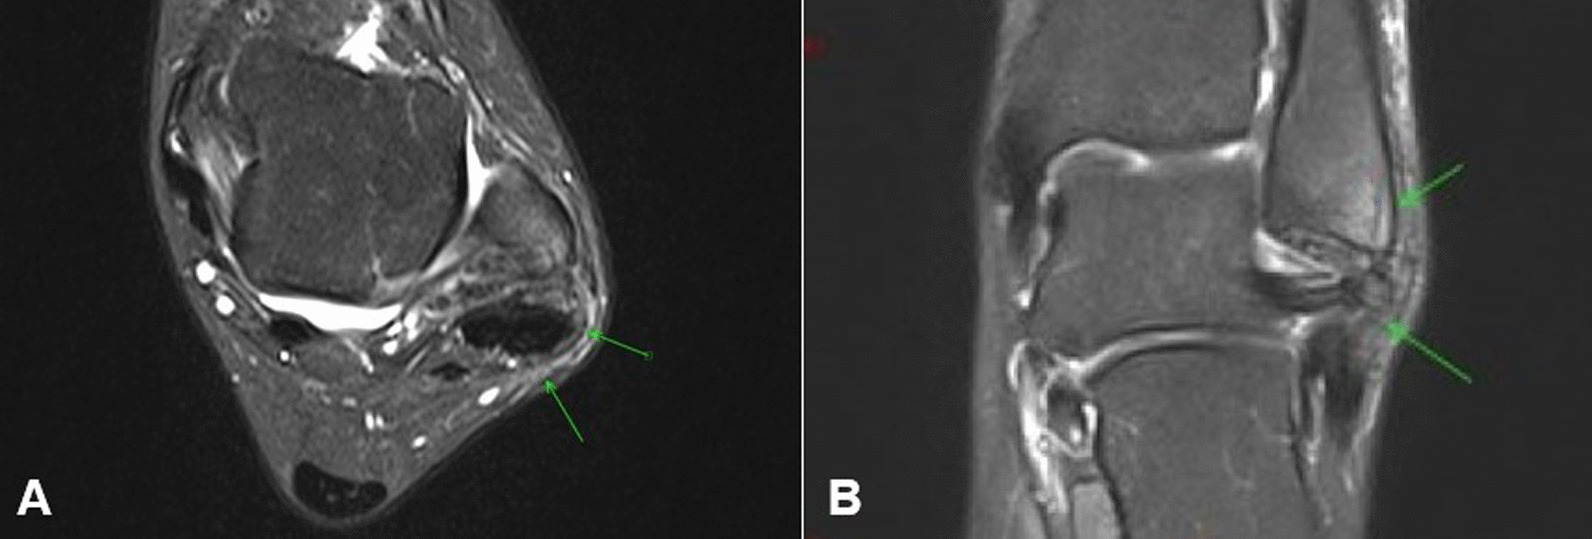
A Axial T2-weighted magnetic resonance imaging of left ankle, displaying repaired retinaculum (green arrows). B Coronal T2-weighted image showing the repaired retinaculum (green arrows)
